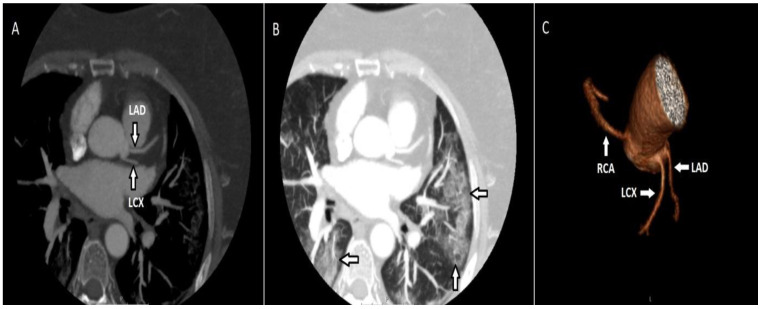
Maximum-intensity projection (MIP) of the axial CT image (A) demonstrates the LCX and LAD emerging from the left coronary sinus with separate ostia. The same patient had ground glass infiltrates consistent with COVID-19 (B). On the volume-rendered (VR) image (C), the LAD and LCX emerge from the left coronary sinus, along with the RCA emerging from the right coronary sinus.
